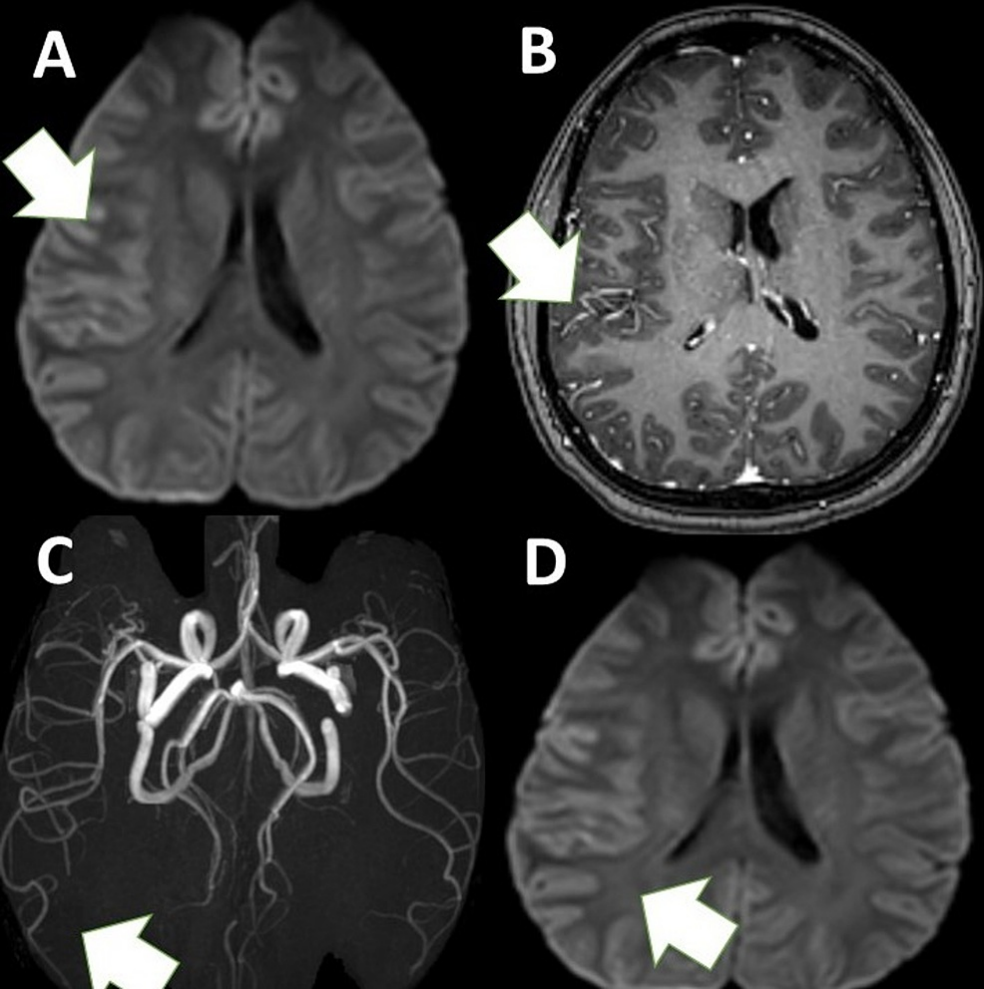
MRI images. (A) Right-sided FLAMES. (B) MRA showing right hemispheric hypervascularity (white arrow). (C) Contrast-enhanced T1-weighted axial image showing focal leptomeningeal enhancement. (white arrow). (D) Diffusion-weighted MRI image showing right hemispheric T2 shine through (no corresponding ADC changes). FLAMES: FLAIR-hyperintense lesions in anti-MOG associated encephalitis with seizures; FLAIR: fluid-attenuated inversion recovery; MRA: magnetic resonance angiography; MRI: magnetic resonance imaging; ADC: apparent diffusion coefficient
